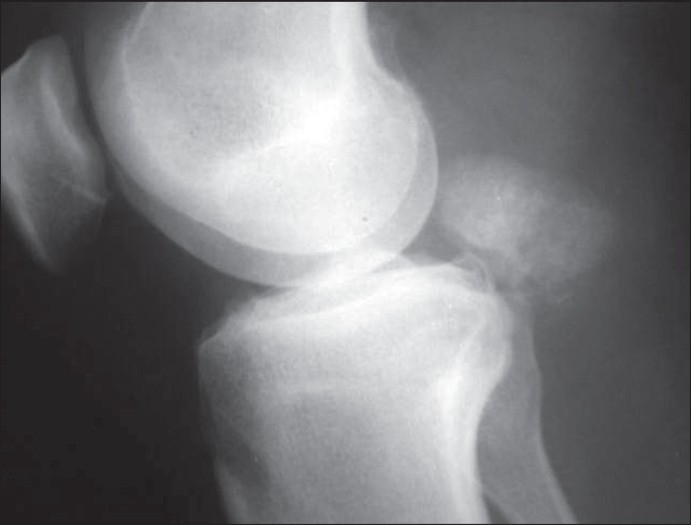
Lateral radiograph of the right knee showing a single calcified body
